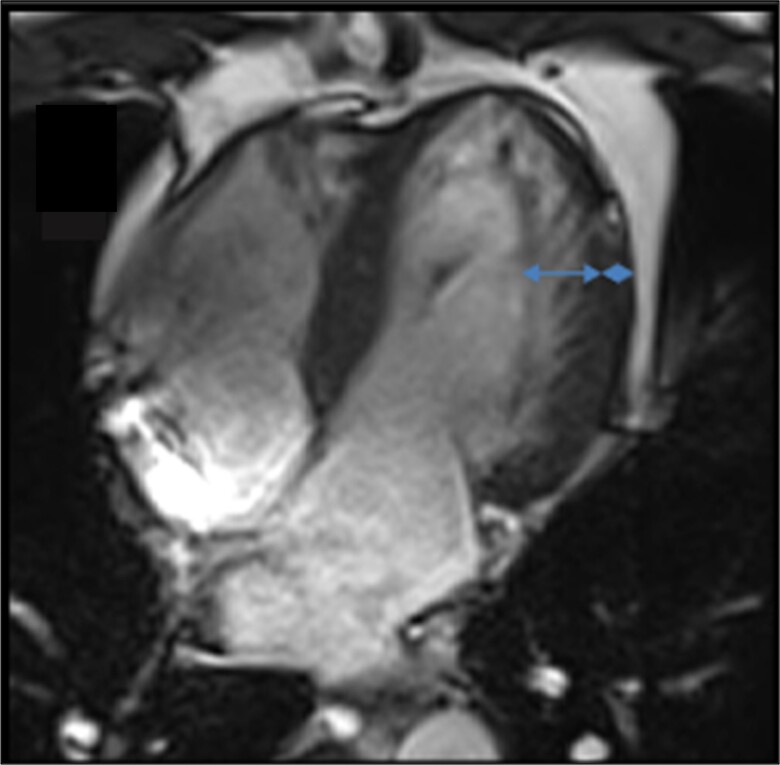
Cardiac magnetic resonance imaging, apical four-chamber view: calculated non-compacted/compacted myocardium ratio over 2.8 depicts left ventricular non-compaction cardiomyopathy involving apical and lateral walls.
